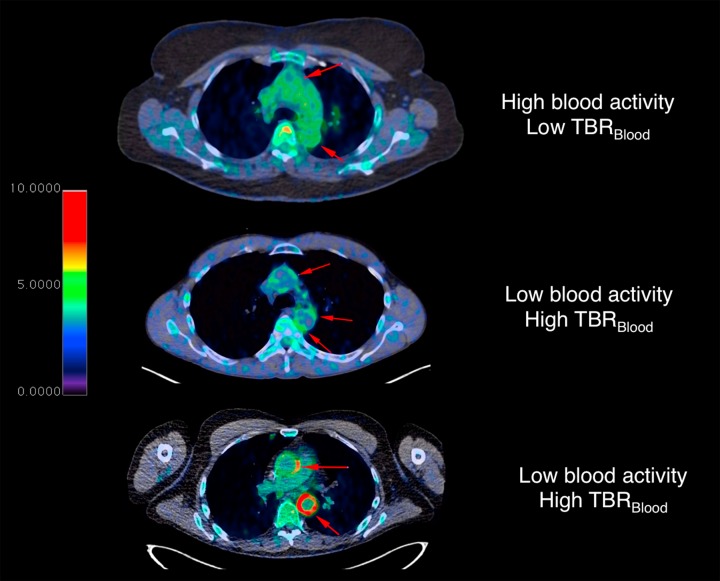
Examples of variation in blood activity and aorta wall FDG uptake. PET (color) and CT (black/white) axial fusion images just below the aortic arch of three subjects, using identical windowing and leveling of PET SUV according to the look up table (left). Red arrows point to regions where aorta wall activity is expected. The top subject has low TBR_Blood_ with high levels of blood activity in the aorta lumen (green). The middle subject has lower blood activity in the lumen of the aorta, which allows visual contrast of the aorta wall, therefore higher TBR_Blood_. It is not clear if the difference in TBR_Blood_ between the top two subjects is related to differences in inflammation or due to differences in blood activity. However, compared to a subject with active large vessel vasculitis (bottom), there is clearly abnormal aorta wall activity that can be distinguished from blood activity.
